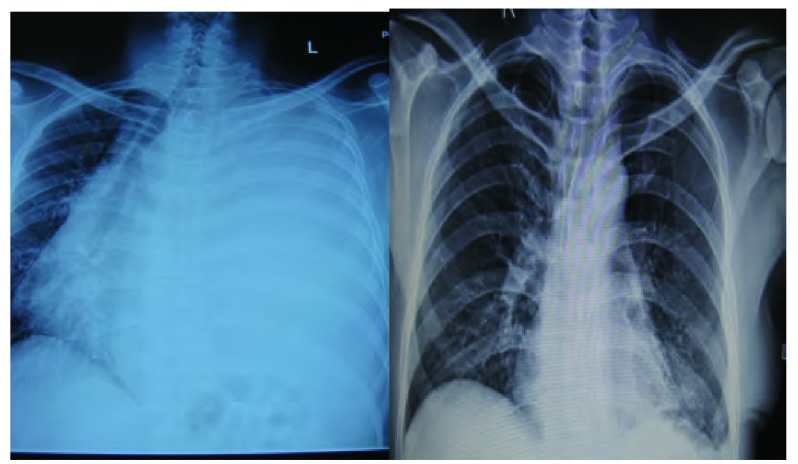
术前胸片，可见纵膈明显向右移位，左侧肺野高密度影。术后复查胸片示肺复张良好，左下可见引流管影。 Chest radiography before surgery shows mediastinum shift to right apparently and high density image in the whole left lung field. However, the left lung-re-expansion well and drainage tube seen in the postoperative chest radiography.
